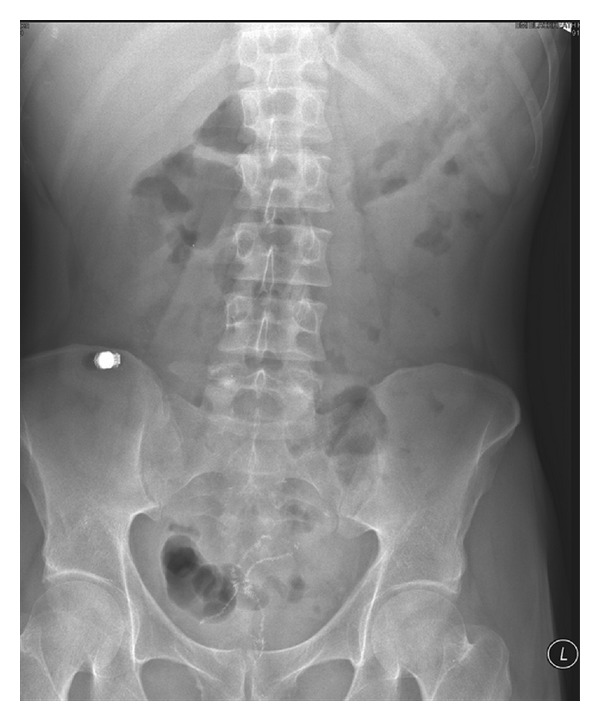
Plain abdominal X-ray showing retained capsule.
